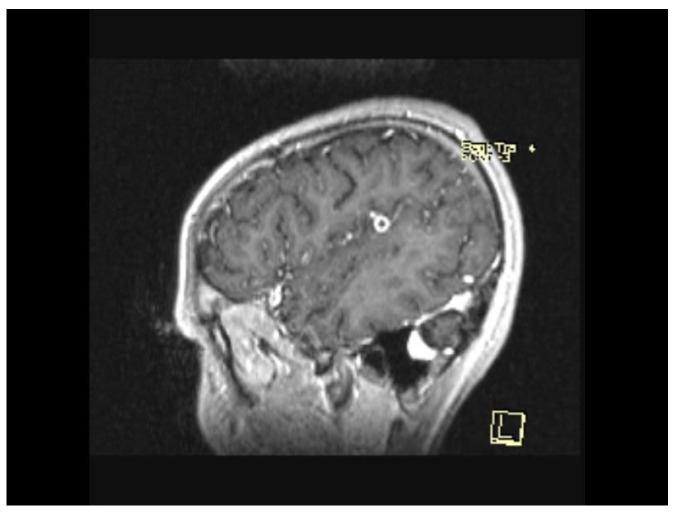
MRI Peripheral annular contrast enhancement, 9/6/6.8 mm in size, with the appearance of a tuberculoma, situated in the left temporal area. The left parietal sequelae, 4/3.8/7.1 mm and 5.1/3.7/5.3 mm in size.
